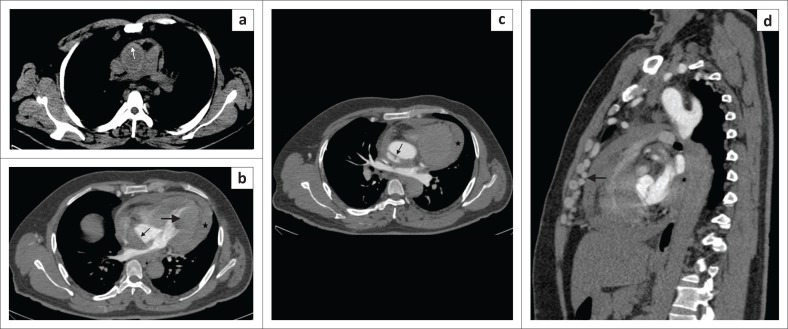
Coarctation of the aorta with a type A intramural haematoma (IMH) and aortic dissection. (a) Unenhanced axial computed tomography (CT) scan showing circumferential hyperdense thickening of the ascending aorta (arrow); (b) Contrast-enhanced axial CT showing crescentic non-enhancing hypodense area (thin arrow) representing IMH with haemopericardium (*) and concentric left ventricular hypertrophy (thick arrow); (c) Contrast-enhanced CT scan showing the displaced intimal flap (arrow) and haemopericardium (*). (d) Sudden narrowing of the aorta (arrow) distal to origin of the left subclavian artery on sagittal contrast-enhanced images. Multiple collaterals (thick arrow) along the anterior chest wall.
